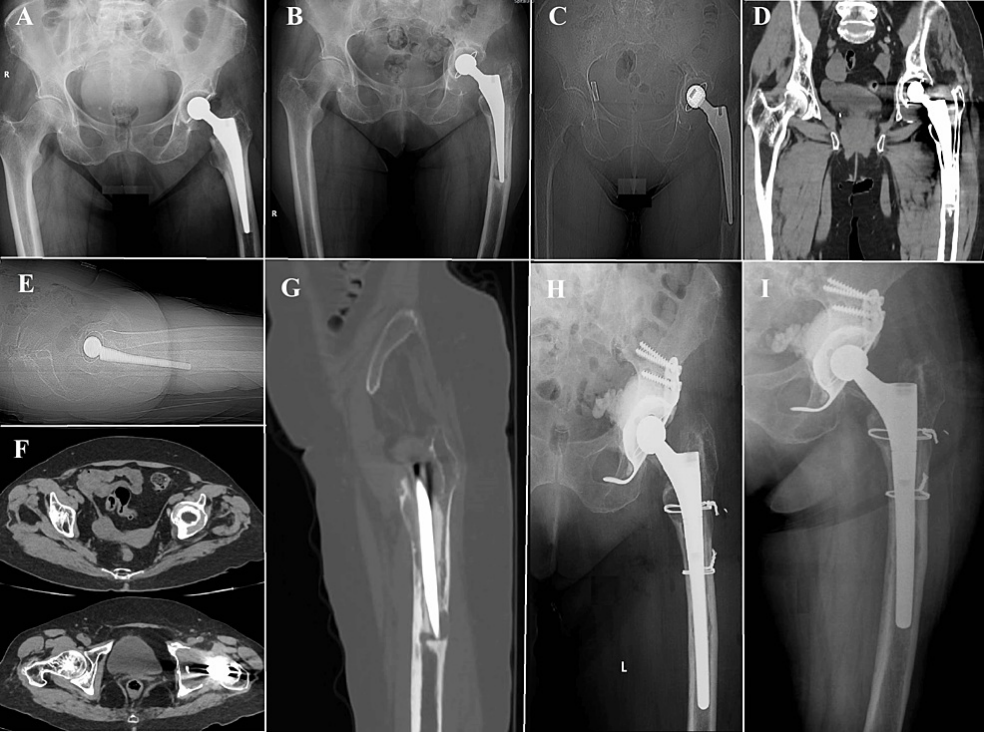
Vancouver B1, Case three (A) Seven years post total hip arthroplasty, slight deterioration; (B) 11 years post arthroplasty, significant deterioration with discomfort; (C-G) CT scans confirm femoral lysis and acetabular loss; (H) Revision hip arthroplasty includes cerclage wires stabilizing the extended stem; (I) One year post-revision, successful healing and prosthesis integration, emphasizing the importance of postoperative monitoring and potential revision procedures for specific fracture characteristics.
